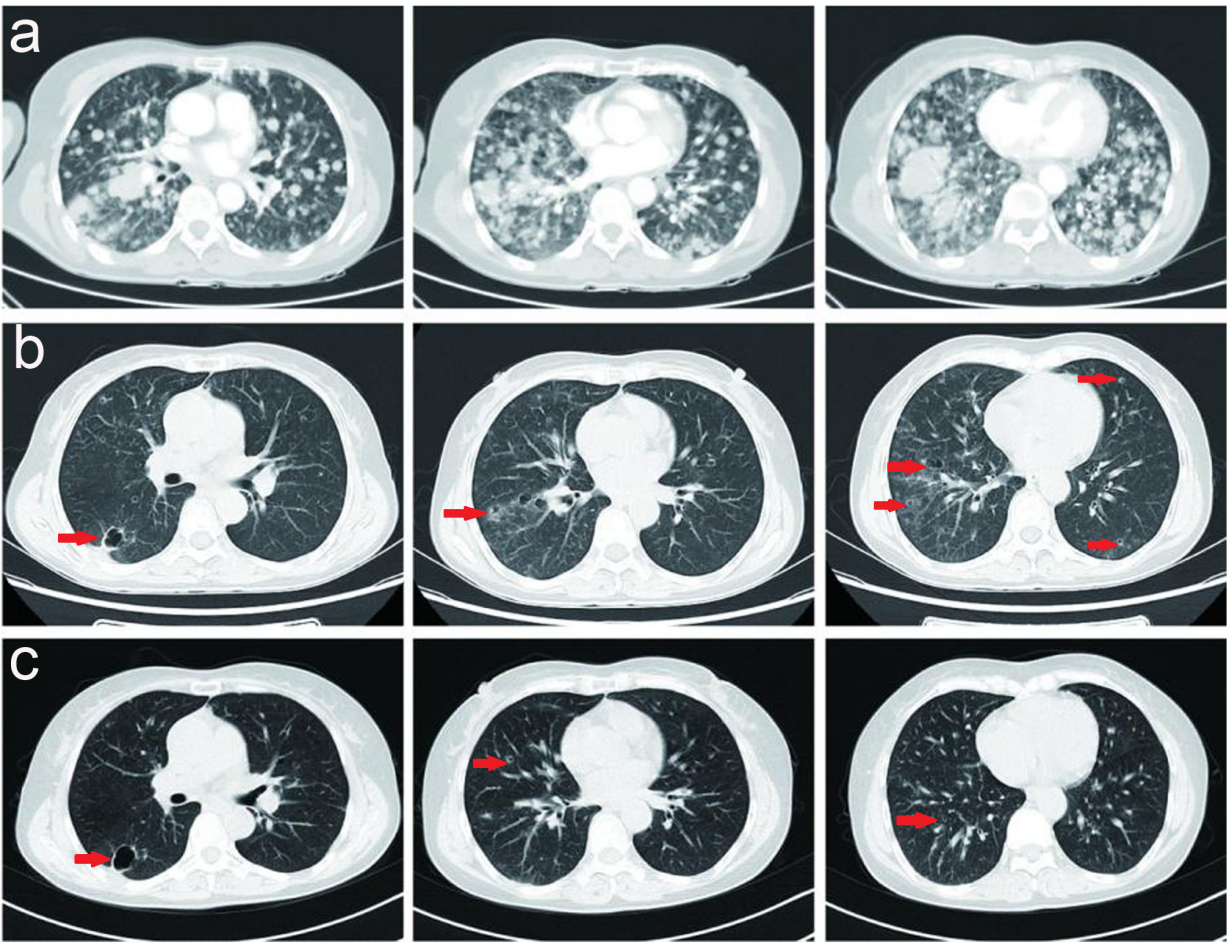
(A) Serial CT scans of a combination of bevacizumab (400 mg, day 1) plus pemetrexed (800 mg, day 1) and carboplatin (400 mg, day 1) for 4 cycles as a first-line treatment, and the tumor burden was progressed. (B, C) Serial CT scans of a combination of bevacizumab (400 mg, day 1) plus abraxane (400 mg, day 1) and nedaplatin (100 mg, day 1) for 3 cycles as a second-line treatment, and revealed prominent regression of primary lung tumors in the right lower lobe and lung-to-lung metastases.
